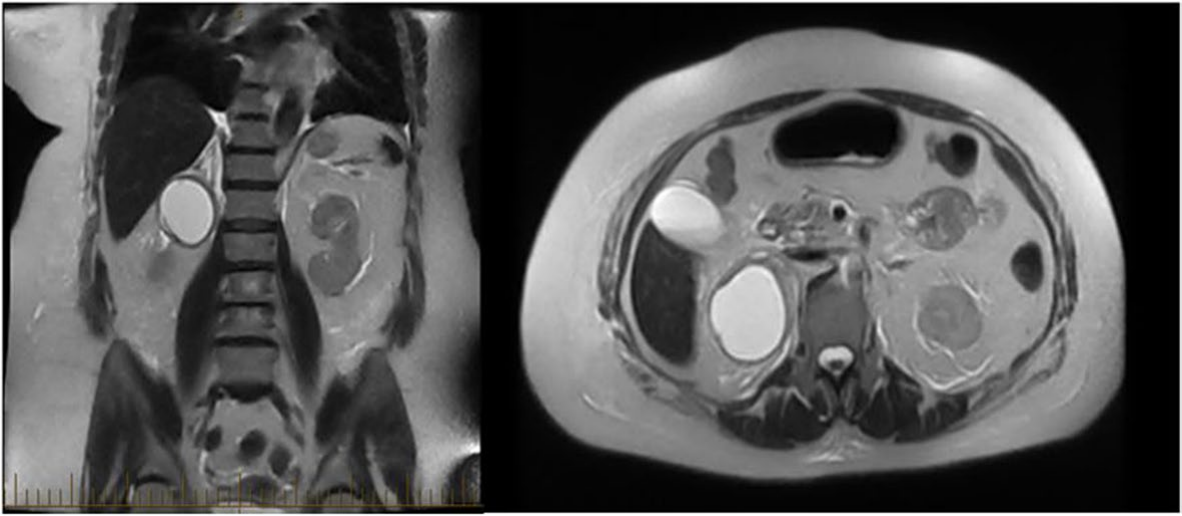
Coronal T2 view of 6.7 cm cystic right adrenal mass on MRI (left) with axial slice on right
